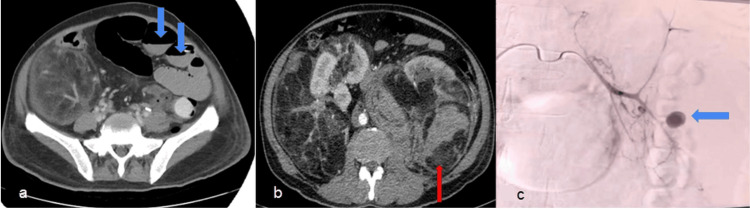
Computed tomography of the abdomen and renal angiogram images AML: Angiomyolipoma (a) Computed tomography abdomen showing the dilated bowel loops (blue arrow) with multiple air-fluid levels indicating subacute intestinal obstruction. (b) Computed tomography of the abdomen showing bilateral renal AML, right inferior pole renal angiomyolipoma, and left renal angiomyolipoma replacing the kidney in its entirety with hematoma in the lower pole (red arrow). (c) Pseudoaneurysm of the left renal artery (blue arrow). Super selective renal artery embolization was done
